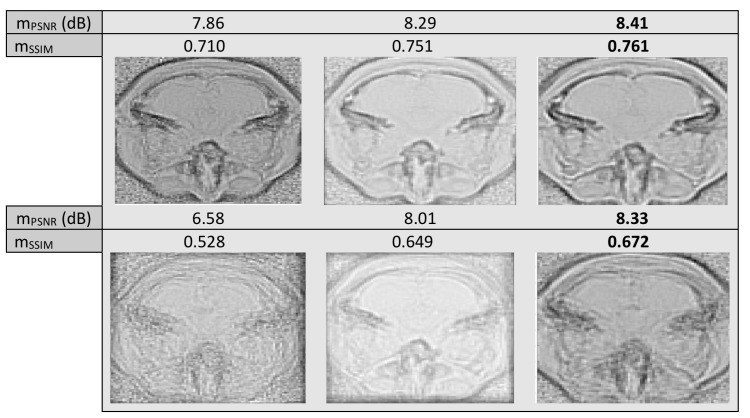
Mean misalignment images of T1, T2 MR images with warp of σ=1 (first row) and σ=5 (second row). Images before alignment (first column) and after alignment with LKE (second column) and the proposed (third column).
